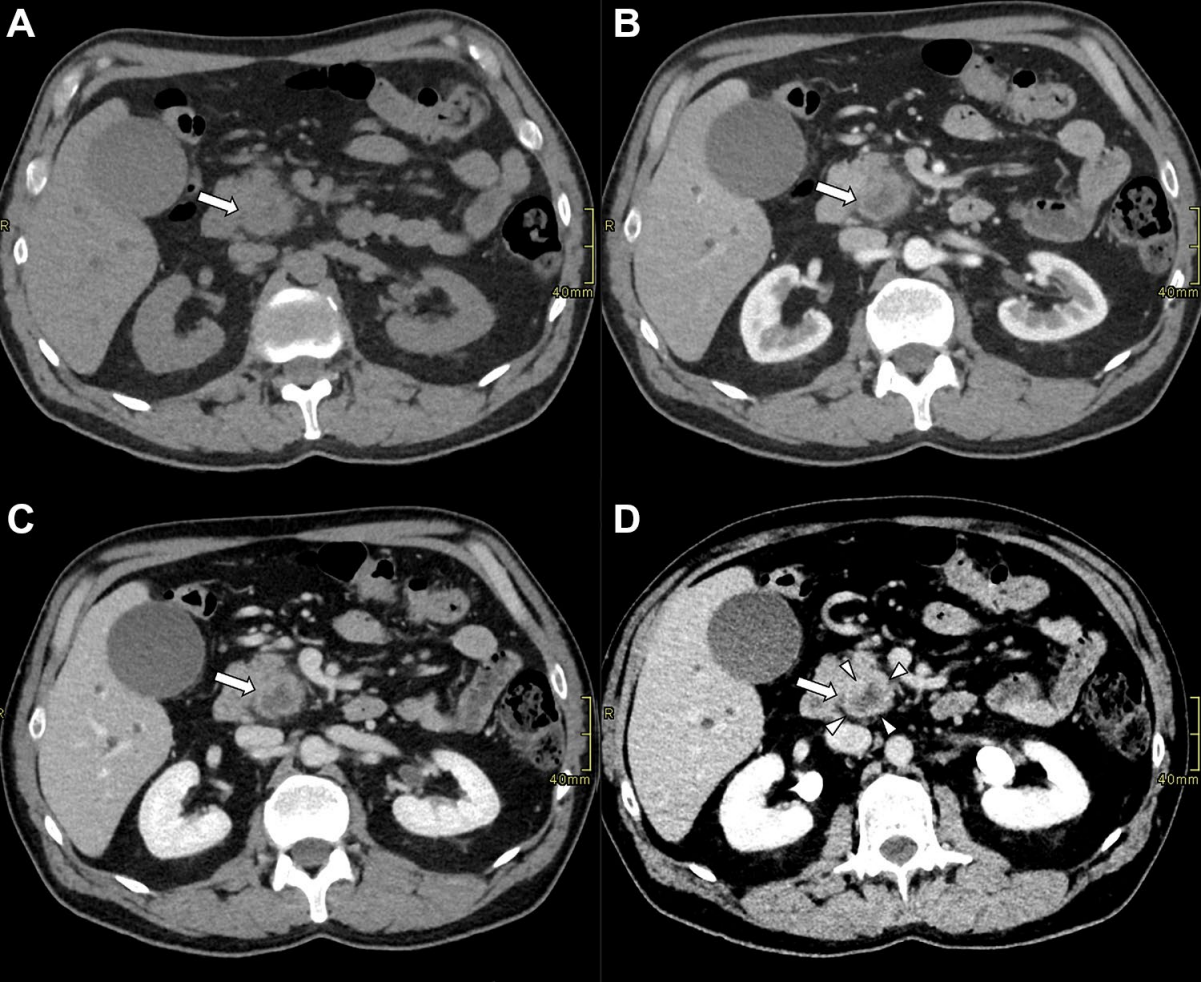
Axial contrast-enhanced CT images of a pancreatic ductal adenocarcinoma at the pancreatic head (arrows), acquired in unenhanced (A), pancreatic (B), portal venous (C), and delayed (equilibrium) (D) phases. In the delayed phase (D), a well-defined enhanced rim (arrowheads) becomes visible, delineating the lesion margin more clearly than in the earlier phases
